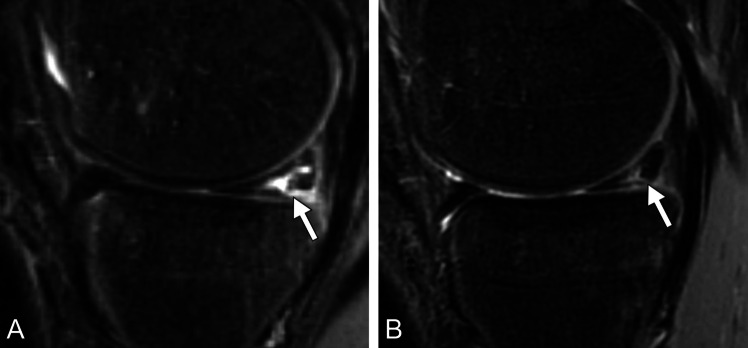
Postoperative meniscus. Preoperative sagittal T2-weighted fat-suppressed MRI (A) of a 22-year-old woman with chronic knee pain demonstrates branching linear fluid signal intensity in the medial meniscus posterior horn (arrow) diagnostic of a complex tear. Sagittal T2-weighted fat-suppressed MRI (B) obtained one year and one month after medial meniscus repair depicts intermediate signal intensity reparative tissue at site of surgery (arrow)
